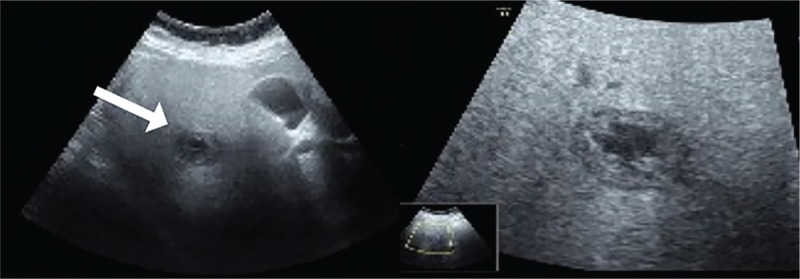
Abdominal ultrasound examination showed uneven fatty liver and a cystic echo mass (white arrow) measuring 30 × 19 × 25 mm^3^, with a hypoechoic nucleus, attached to the inferior surface of the right hepatic lobe.
